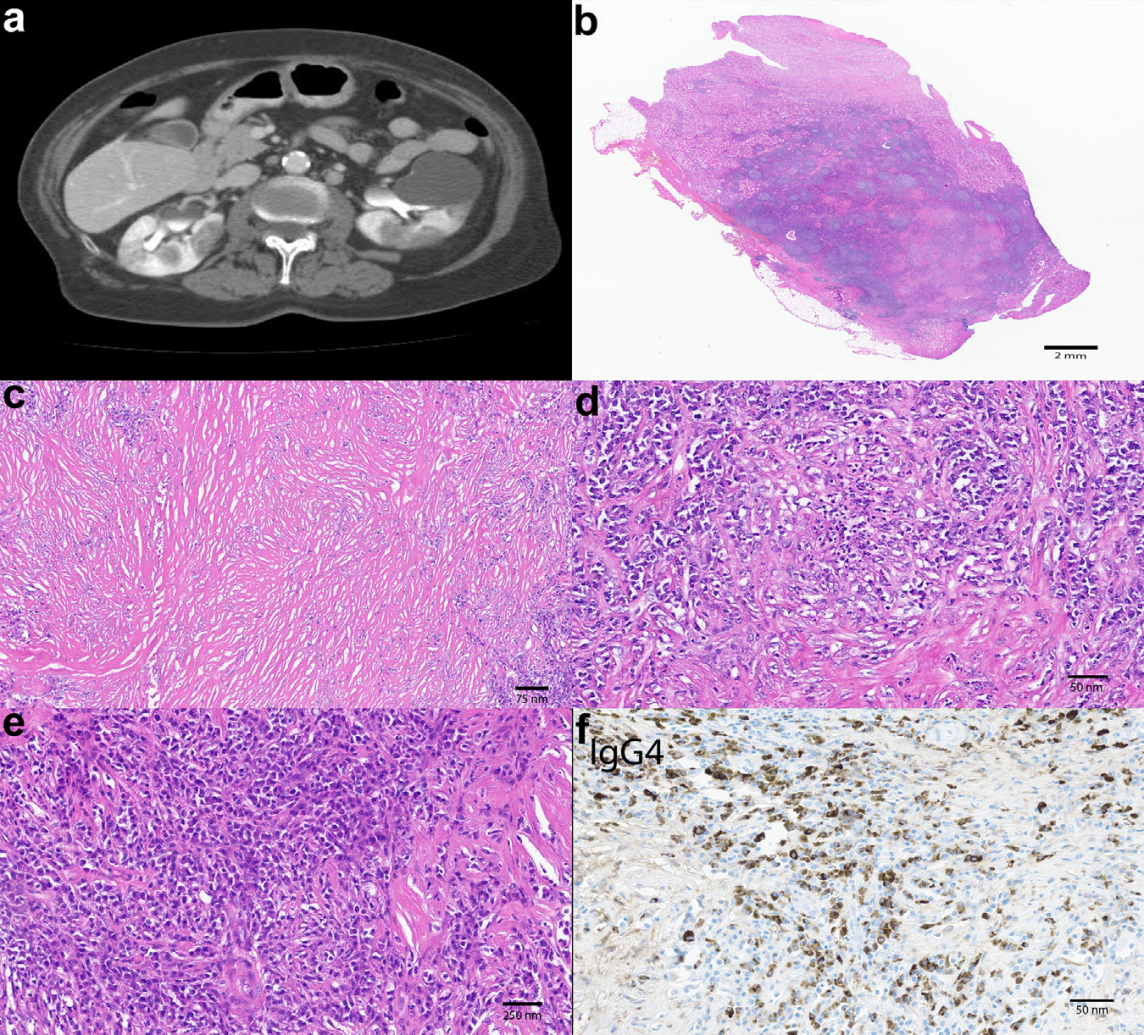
(a) Bilateral renal masses with low to intermediate density on computerized tomography (CT) imaging. (b) Renal parenchyma showing fibrosis and dense chronic inflammation and lymphoid follicles (Magnification: 0.3×). (c) Low power view shows storiform fibrosis and inflammation (magnification: 100×). (d) Intermediate power view showing focal karyorrhectic debris intermixed with interstitial inflammatory infiltrate (magnification: 200×). (e) Interstitial inflammatory infiltrate with predominant plasma cells (magnification: 250×). (f) Intermediate power view showing increased IgG4+ plasma cells in the interstitial inflammation (magnification: 200×).
